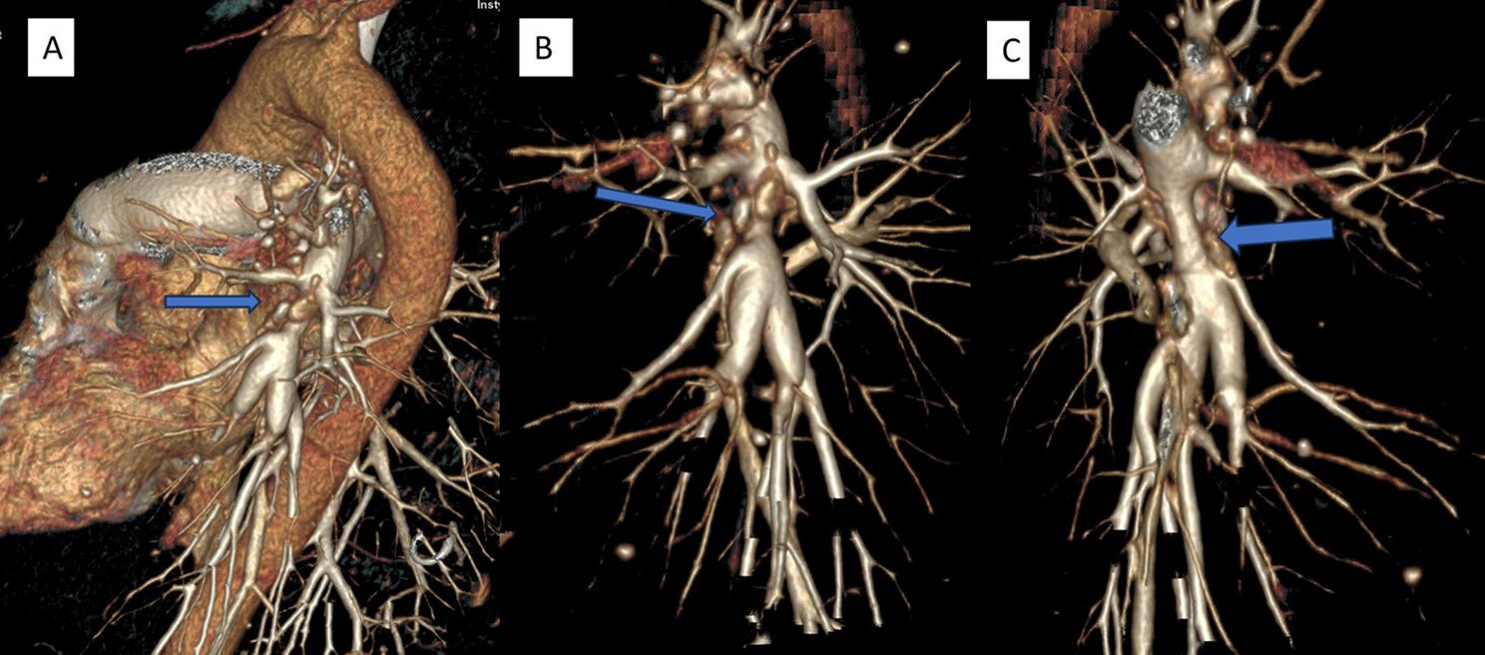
Computed tomography pulmonary angiography, 3D reconstructions precisely show narrowing of the left pulmonary artery and adjacent calcified lymph nodes (blue arrows) (A) lateral view (B) oblique view, and (C) posterior view
